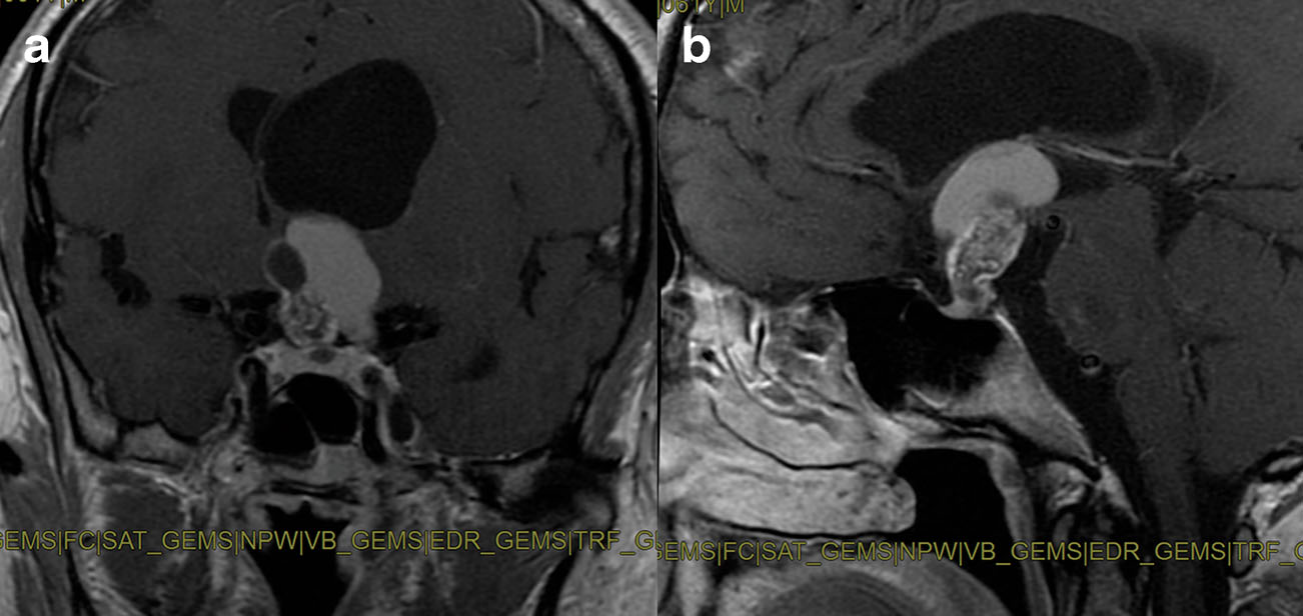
Post-contrast T1-weighted brain MRI, coronal (a) and sagittal (b) views, showing a suprasellar craniopharyngioma with a large third ventricular extension
